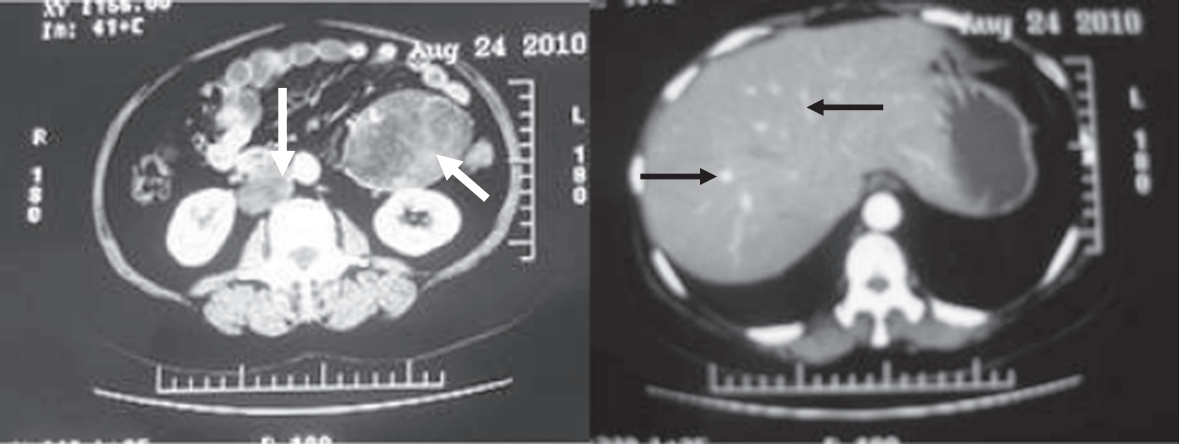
Enhanced abdominal CT scan showing two masses (white arrows), multiple intrahepatic nodules (black arrows) and retroperitoneal enlarged lymph nodes, all enhanced with contrast media.
